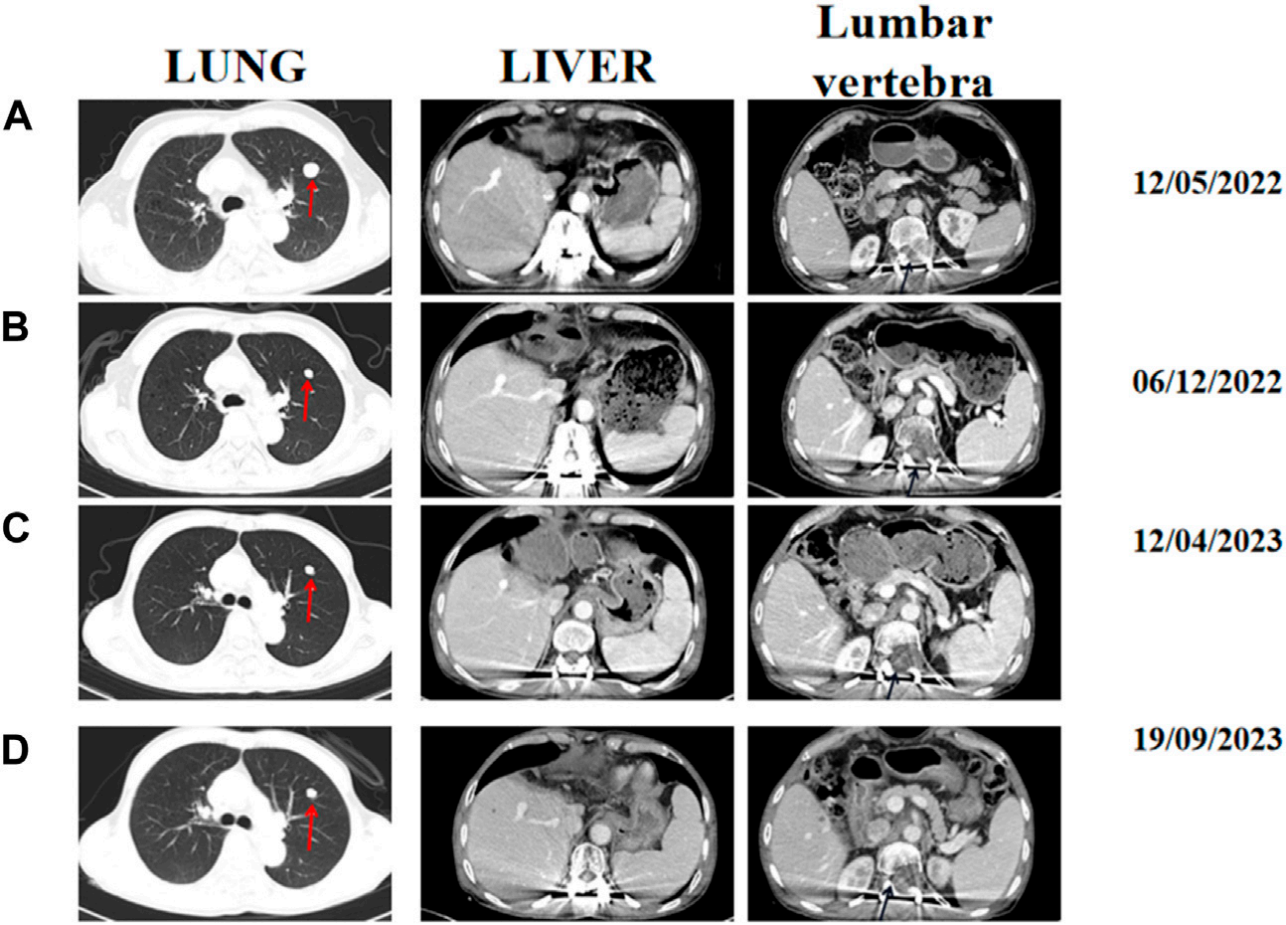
Representative abdominal and chest-enhanced CT images acquired at different times during treatment. (A) Baseline CT images acquired on 12 May 2022. (B) CT image assessment performed on 6 December 2022. (C) CT image assessment performed on 12 April 2023. (D) CT images acquired on 19 September 2023. The red arrows indicate lung lesions, and black arrows indicate lumbar soft tissue.
